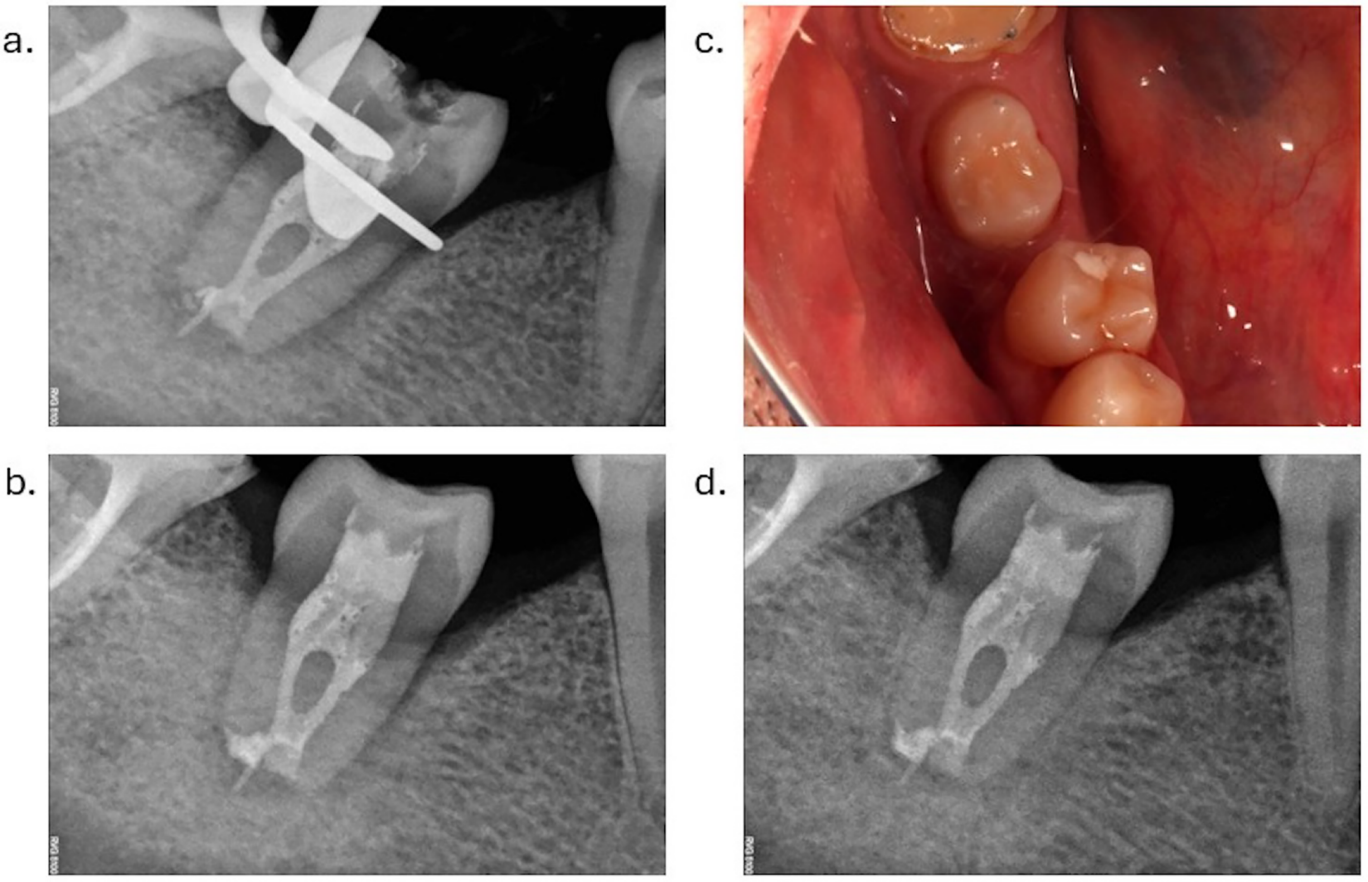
Tooth monitoring following autotransplantation procedure for 10 months. (a) Radiograph with endodontic treatment, one month after autotransplantation. Reorganization of the periodontal space can be observed. (b) Radiograph three months after autotransplantation, with visible narrowing of the periodontal space. (c) Occlusal view of the donor tooth ten months after autotransplantation. (d) Radiograph ten months after autotransplantation, with a normal width of the periodontal space.
